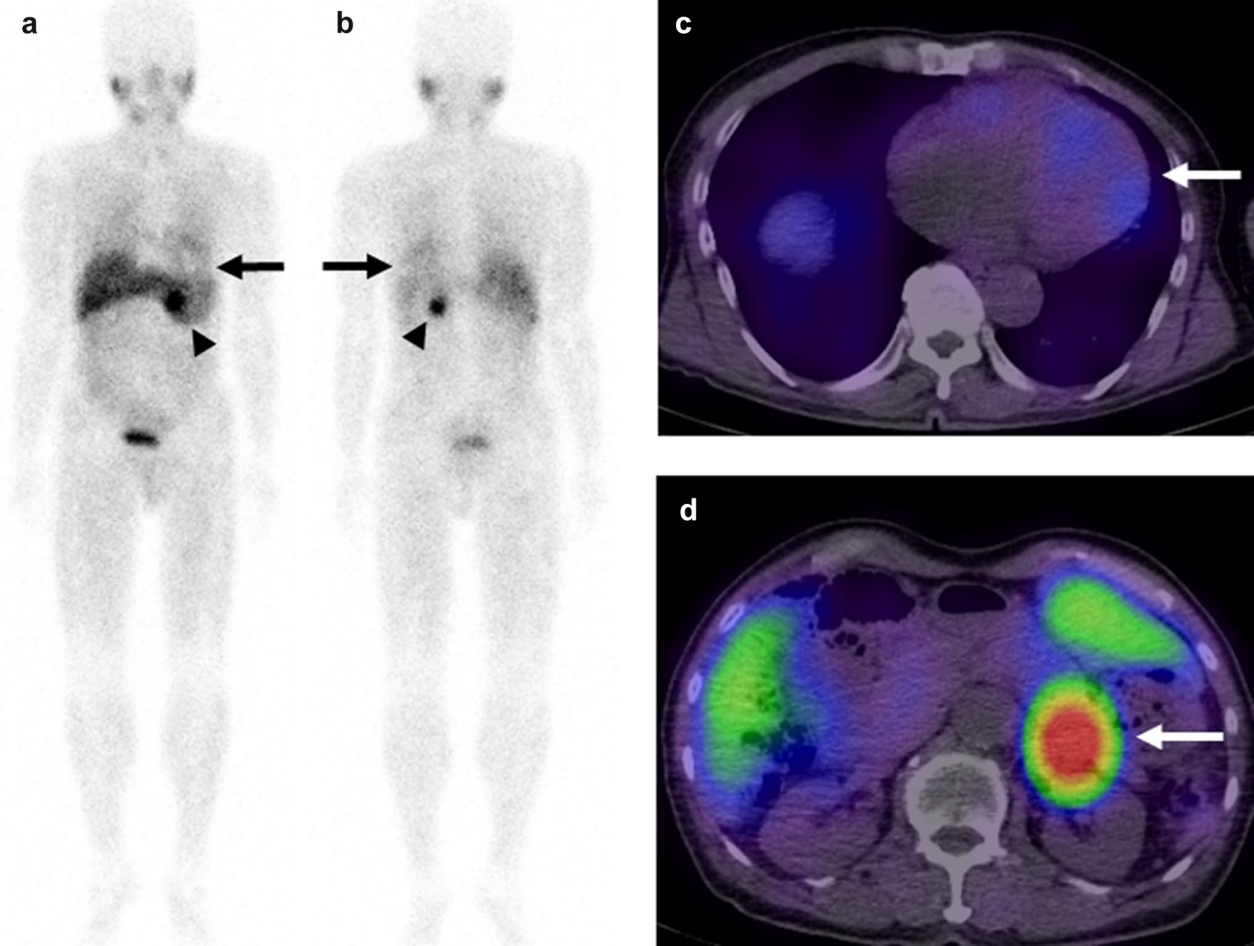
A 65-year-old male patient with left adrenal pheochromocytoma with LV diastolic dysfunction. The [^123^I]-MIBG anterior (a) and posterior (b) planar images reveal decreased cardiac uptake (arrow, score 1) and intense pheochromocytoma uptake (arrowhead, score 3). The [^123^I]-MIBG SPECT/CT images show slight [^123^I]-MIBG uptake in the myocardium (c, arrow), and intense [^123^I]-MIBG uptake in the pheochromocytoma (d, arrow). The myocardium SUVmax, H/M ratio, and pheochromocytoma SUVmax were 1.96, 1.98, and 15.47, respectively. The LVEF, LAVI, E/A, septal e′, and septal E/e′ ratio were 80.0%, 40.6 mL/mm^2^, 0.72, 4.0 cm/s, and 14.2, respectively. These echocardiographic findings indicate the presence of LV diastolic dysfunction
